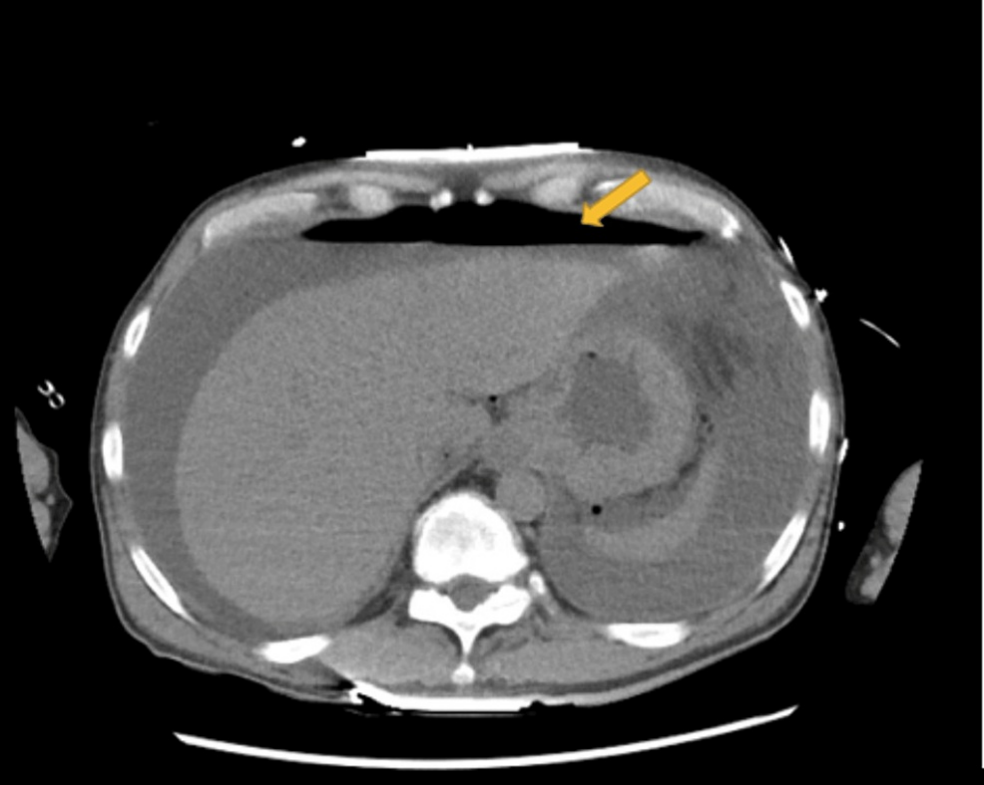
CT scan of the abdomen and pelvis showing pneumoperitoneum (yellow arrow)
